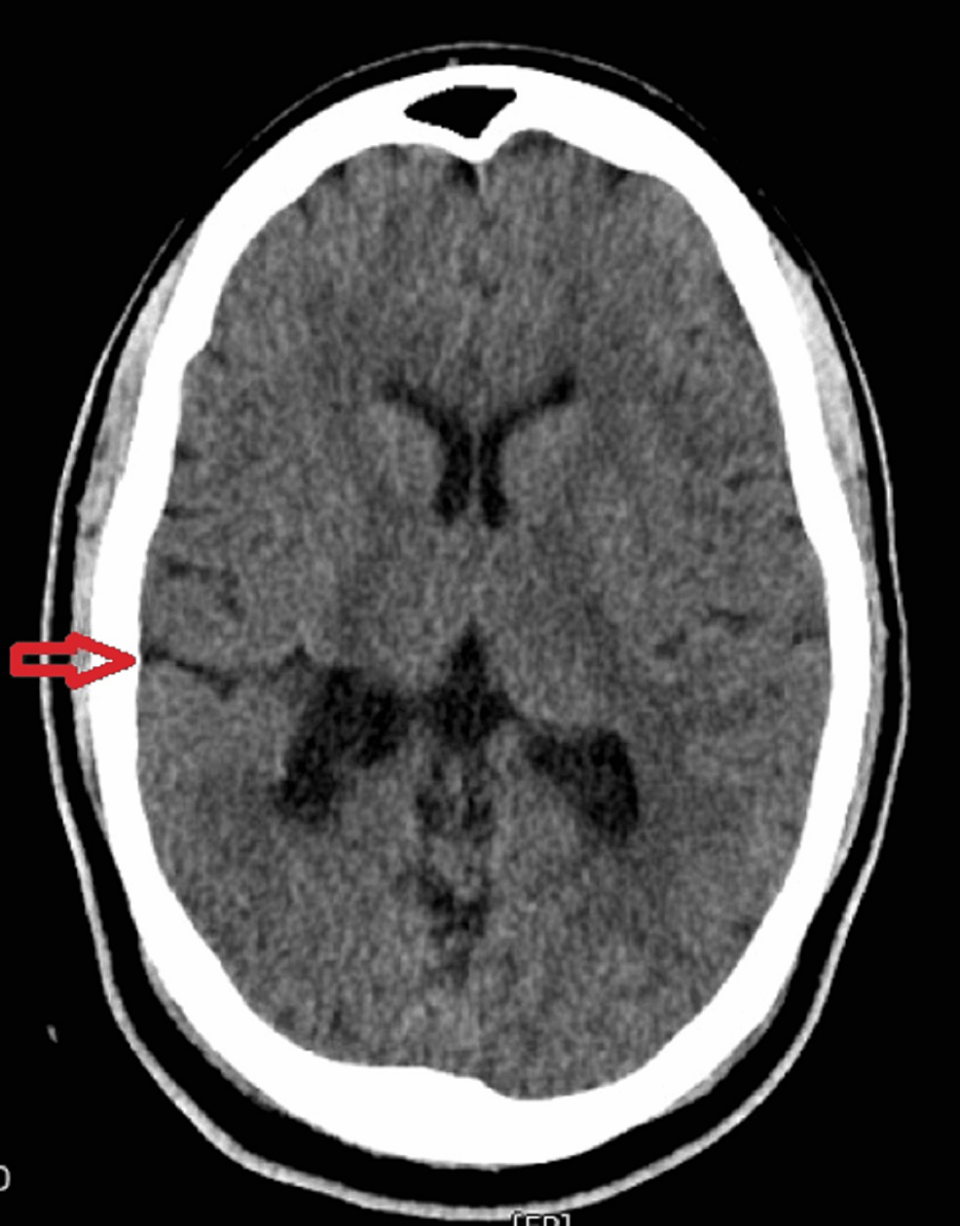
CT of the head without contrast identified open-lip schizencephaly on the right (red arrow) with possible superimposed periventricular leukomalacia adjacent to the right atria.
